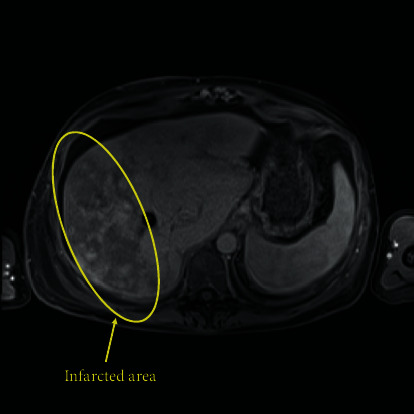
Preoperative MRI demonstrating multifocal infarction of the right lobe of the liver, with TIPs in place.
